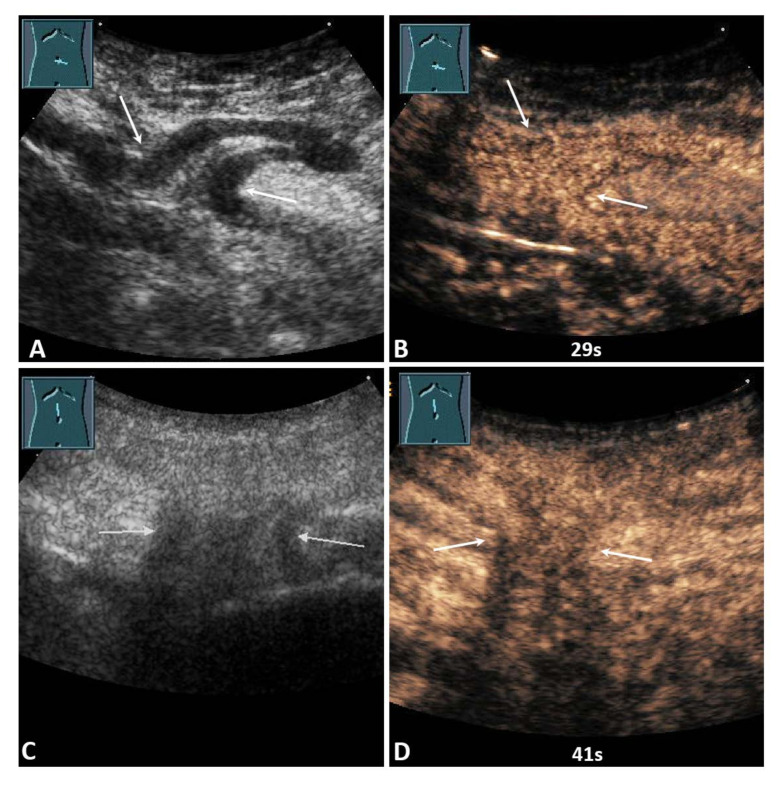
(A) A 59-year-old male patient with acute pain in the paraumbilical region. B-mode ultrasound shows a hernia orifice (arrows), and the hernia sac shows a fixed bowel structure. (B) On contrast-enhanced ultrasound, the loop of small bowel shows a marked enhancement after 29 s. Surgical evaluation showed no evidence of infarction. No resection of the bowel was performed. (C) A 91-year-old female patient with acute pain in the epigastric region. B-mode ultrasound shows a hernia orifice (arrows), and the hernia sac shows fixed omental tissue. (D) On contrast-enhanced ultrasound, the hernia contents show a marked enhancement after 41 s. Surgical evaluation showed no evidence of infarction. No resection of the omentum was performed.
